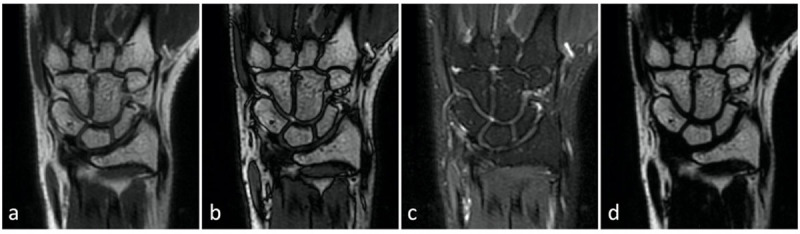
Coronal T2-weighted Dixon images of the right hand in a healthy volunteer. Acquisition of (a) in-phase and (b) out-of-phase images allows secondary reconstruction of (c) fat-suppressed and (d) water-suppressed images by post-processing.
